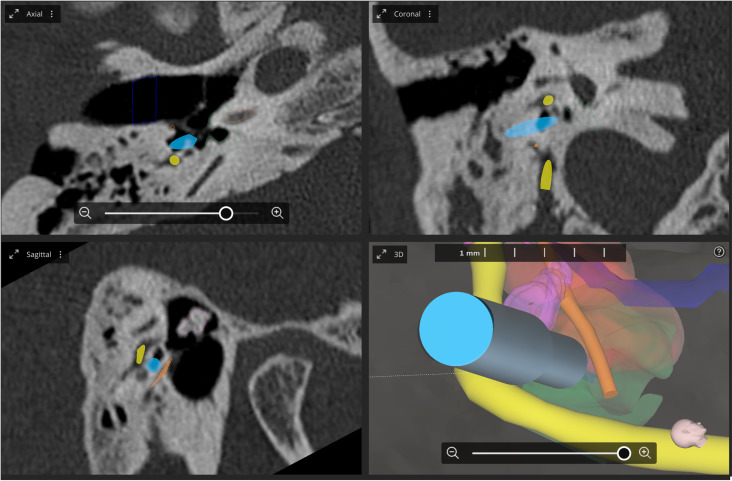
Intra-operative cone-beam CT and 3D reconstruction of incorrectly partially segmented facial nerve (yellow). The drilling trajectory (light blue) was in reality closer to the facial nerve than initially calculated.
